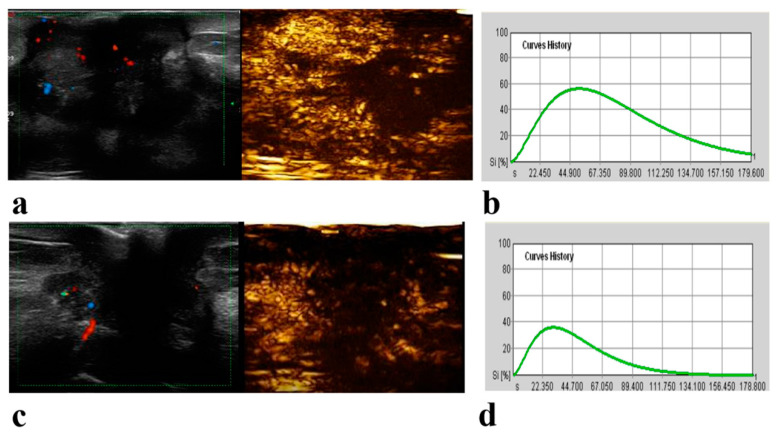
A 56-year-old woman with 4.6 cm luminal B breast cancer of the left breast following neoadjuvant chemotherapy (NAC). Before NAC, Color Doppler US and contrast-enhanced US (CEUS) images obtained 54 s after contrast agent injection show a hyper-enhanced lesion with local large patchy perfusion defect and an indistinct margin (a). Time-intensity curve (TIC) analysis shows peak intensity (PEAK) is 57.60; time to peak (TTP), 50.90 s; regional blood volume (RBV), 6041.92; regional blood flow (RBF), 72.75, and mean transit time (MTT), 83.05 s (b). After two cycles of NAC, Color Doppler US and CEUS images obtained 35 s after contrast agent injection show an iso-enhanced lesion with local large patchy perfusion defect and an indistinct margin (c). The TIC shows PEAK is 37; TTP, 29.95 s; RBV, 2360.43; RBF, 46.84, and MTT, 50.39 s (d). After surgery, pathological analysis showed only a few scattered tumor cells remained in the breast. This patient was found to have contralateral axillary metastasis after a follow-up of 67 months.
